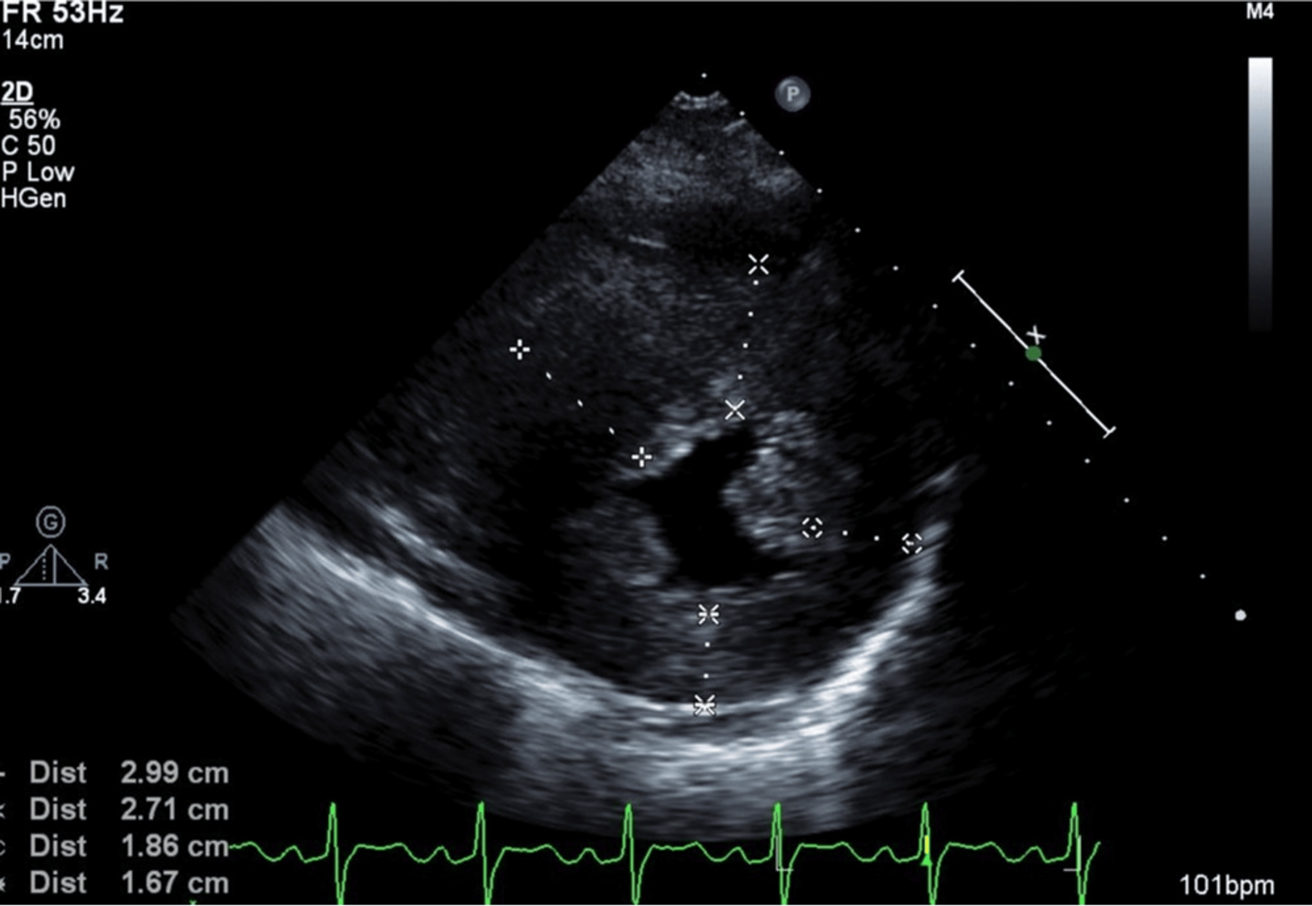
Echocardiography during the course of the first pregnancy The thickness of the left ventricular wall had reached almost 30 mm.
